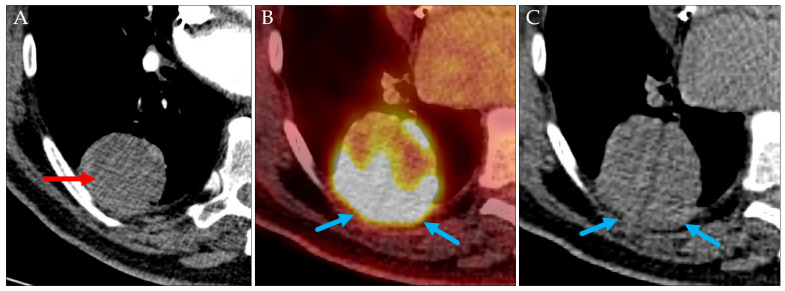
The initial CT (A; axial, contrast-enhanced) revealed a 5.2 cm mass in the right lower lobe abutting the pleura (red arrow; cT3). Axial PET/CT (B) and CT (C) performed 28 days later showed size progression to 6.4 cm and infiltration of the parietal pleura (blue arrows, still cT3).
